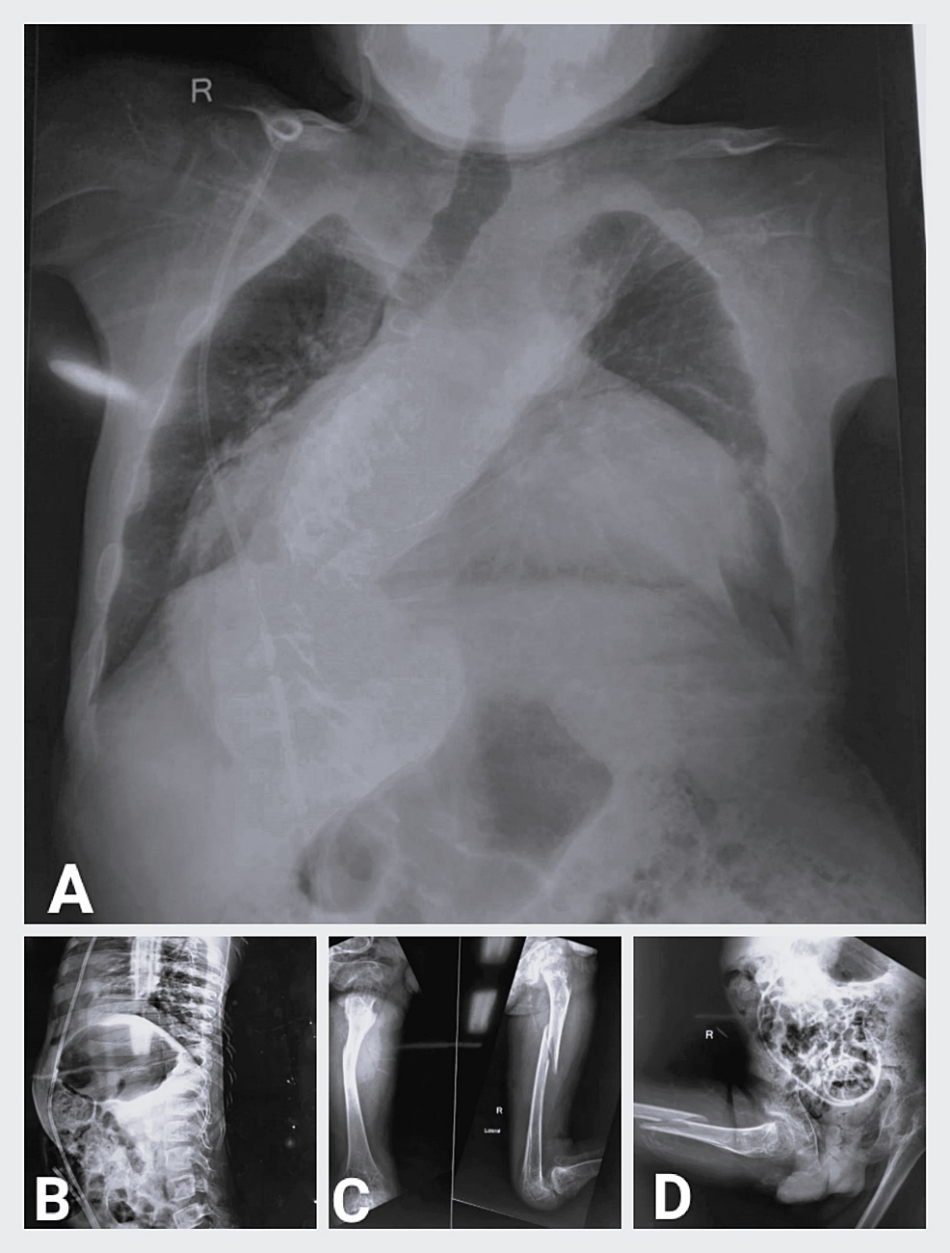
X-ray films of the chest, spine, femur, and pelvis (A) X-ray films of the chest showing scoliosis and deviated trachea toward the right. (B) X-ray thoracolumbar spine with abdomen showing ventriculoperitoneal shunt in situ. (C) X-ray anteroposterior and lateral view of the right femur showing femur shaft fracture. (D) X-ray pelvis with bilateral hip showing pelvic deformity along with ventriculoperitoneal shunt in situ and right femur shaft fracture
